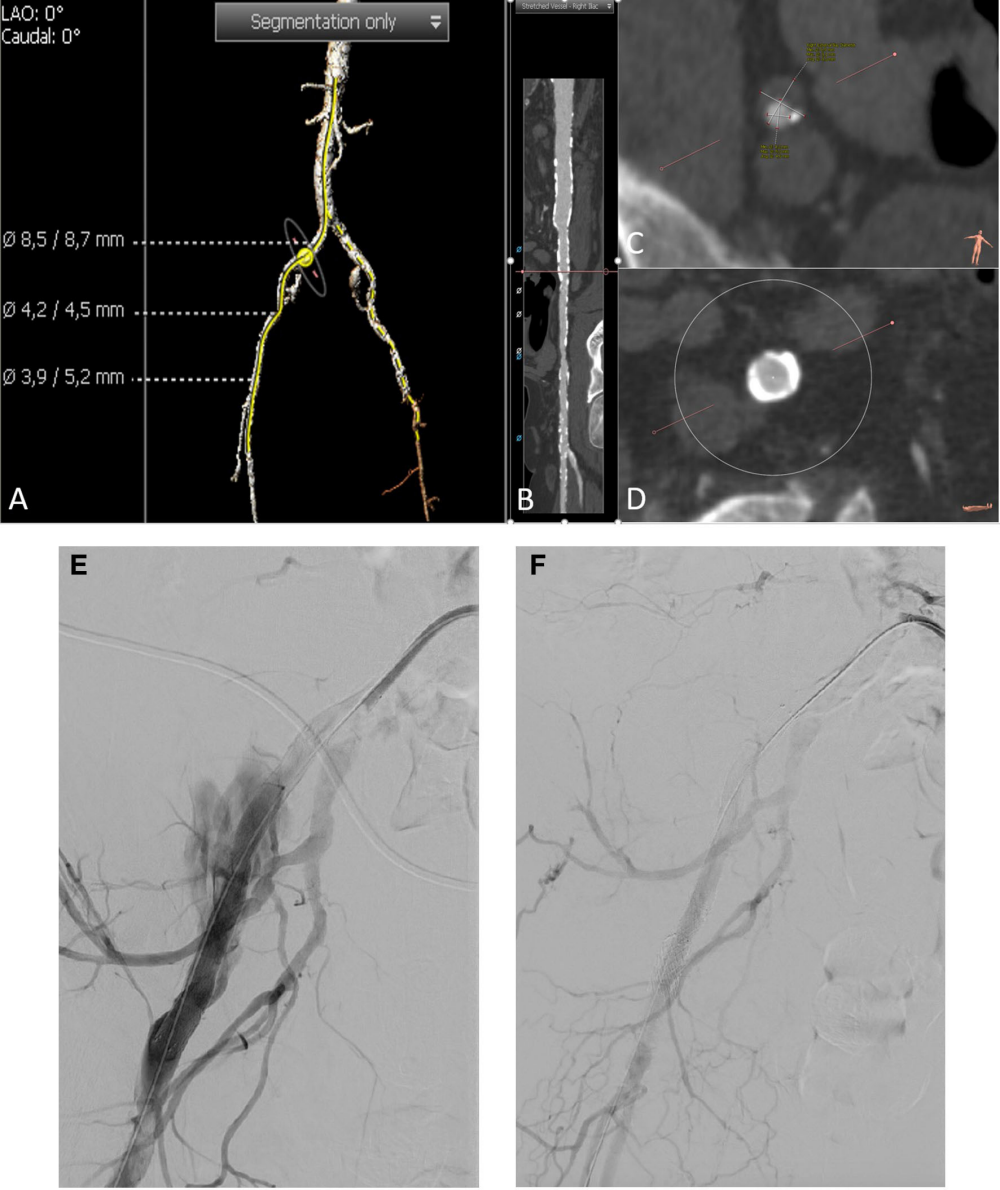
A CT angiography unraveled severely calcified iliofemoral arteries (A–D). Three-dimensional reconstruction of pelvic arteries with the measurement of common femoral artery diameter, minimal external iliac diameter, and common femoral arterial diameter (from up to down) (A). A stretched vessel analysis of the right pelvic axis shows severe calcification of the vessel (B). Minimal diameter of the right external iliac artery is 4.2 mm (C). Right common iliac artery with circular calcification (D). After initial discussion of a pre-TAVI vessel preparation with by lithoplasty according to the CT angiography, we decided to go on without any peripheral intervention before TAVI. The balloon valvuloplasty of the aortic valve was performed without any complications. Afterward, we were not able to pass the new valve (CoreValve™, Medtronic, Ireland) through the iliac artery on the right side. Thus, we decided to upsize the sheath to 18 F (Sentrant™, Medtronic), again we were not able to pass the iliac arteries. Another angiography showed a perforation of the right external iliac artery (E). We immediately blocked the bleeding site with an 8.0 mm × 80 mm balloon (Charger, Boston Scientific, MS, USA). We now implanted an 8.0 mm × 100 mm as well as a 8 F compatible 11 mm × 39 mm balloon expandable covered stent prothesis (Gore® Viabahn® VBX, Gore) which finally fixed the perforation (F).
